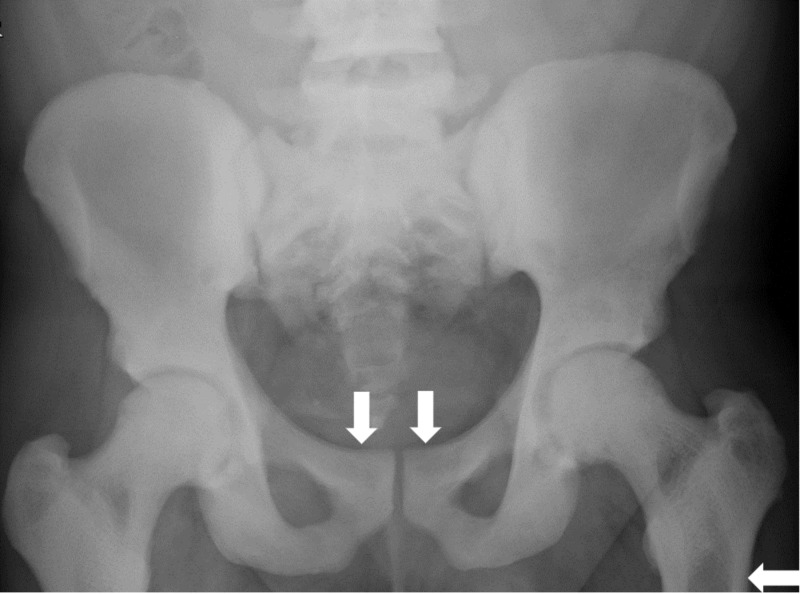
Pelvic radiograph Diffuse increased density of visible bones with smooth, symmetric periosteal reaction along the superior pubic rami and subtrochanteric femurs.
